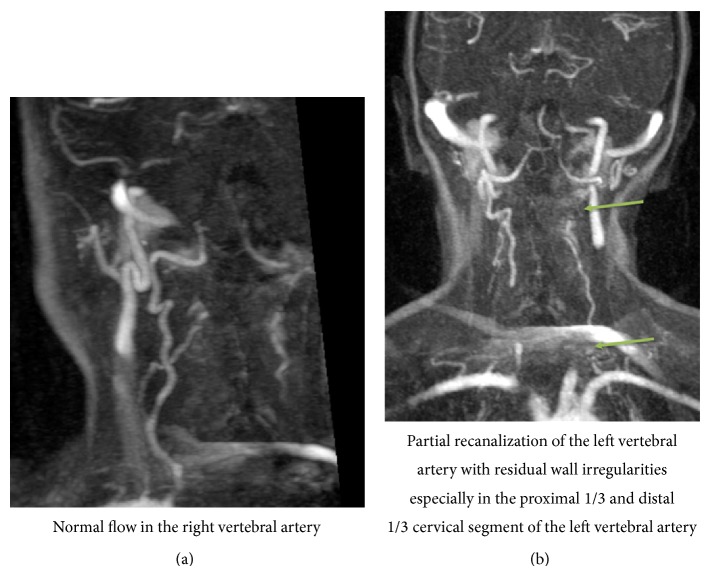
MRI angioscan (FLASH 3D coronal images after Gd-chelate administration).
